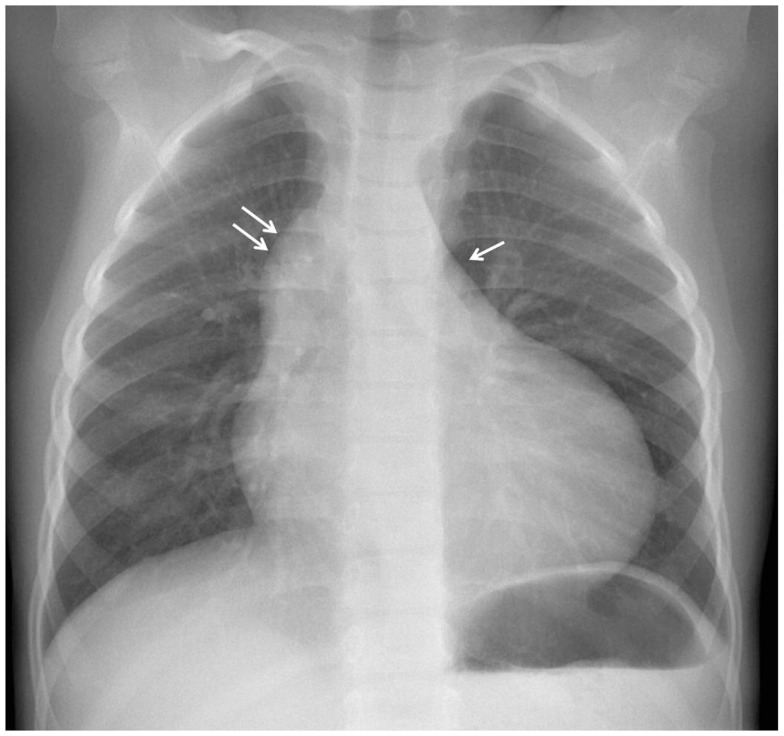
Chest X-ray with prominent right cardiac border secondary to dilatation of the ascending aorta (double arrow) and absent aortic knob (arrow).
